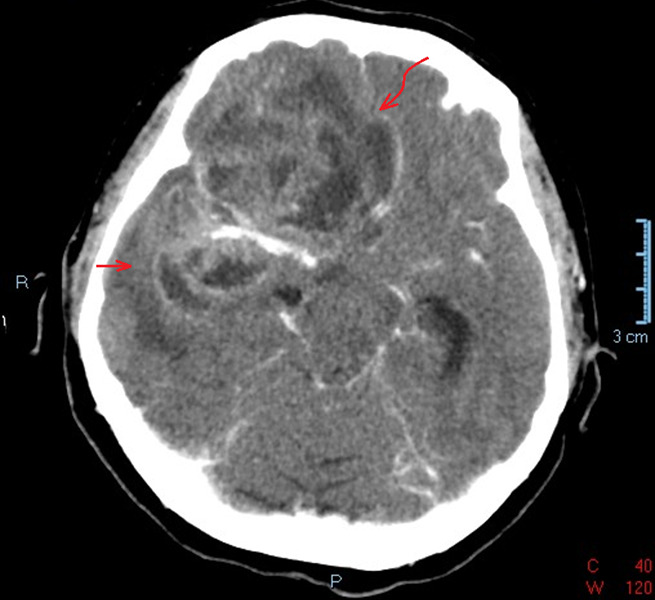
Preoperative CT The tumor is highlighted invading the frontal and temporal lobes as well as the head of the caudate nucleus, the internal capsule and the lenticular nucleus, surrounded by edema (straight arrow) and shifting the midline (undulated arrow).
